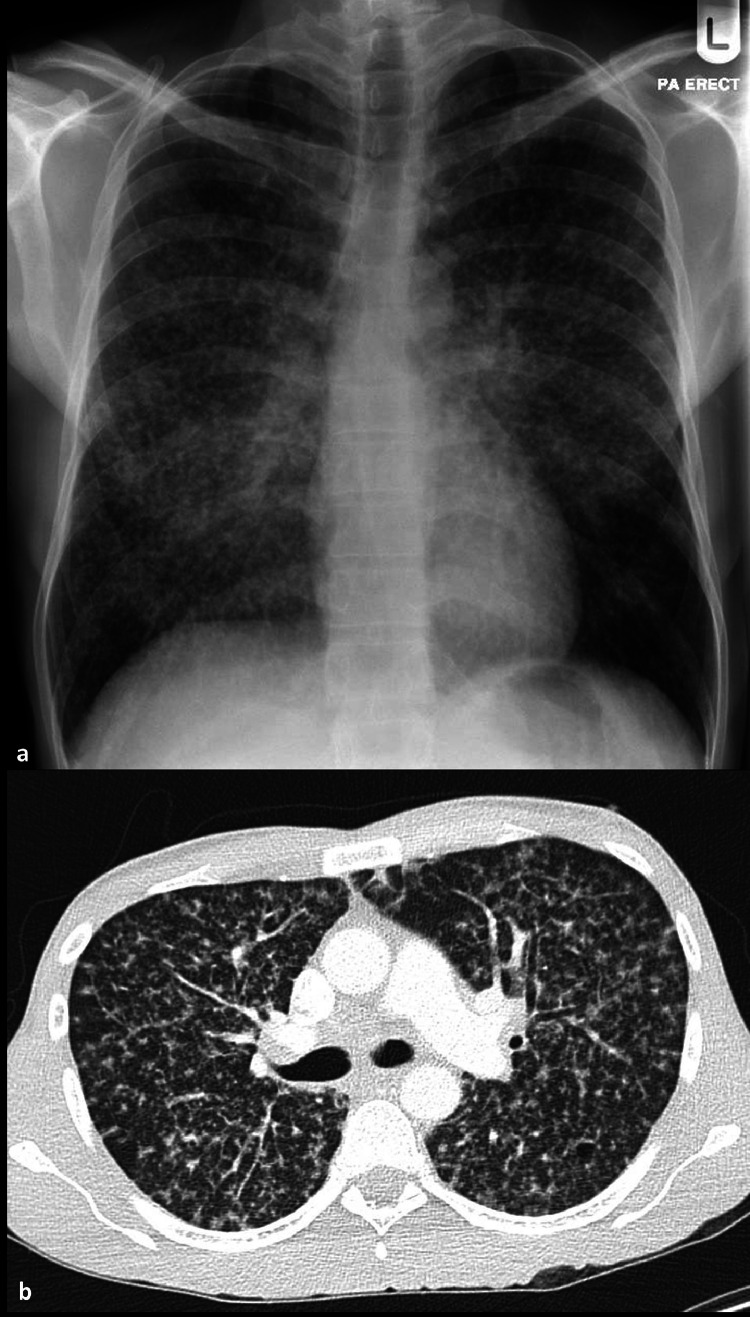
Radiological images from a patient with pulmonary histoplasmosis. (a) Chest X-Ray and (b) computed tomography. The radiological appearance mimicks that of miliary tuberculosis.
